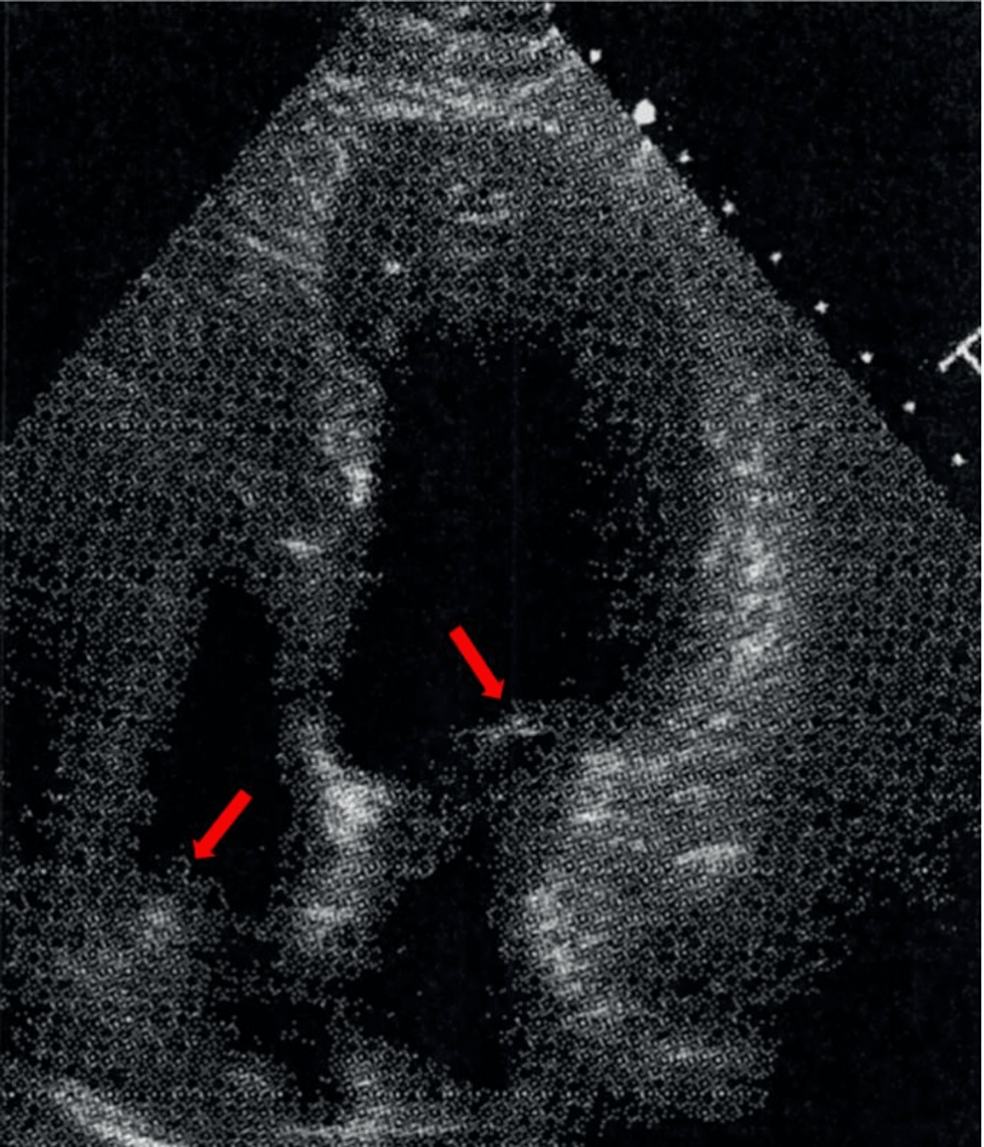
An echocardiogram image of the patient with mildly enlarged left ventricular (LV) wall thickness and pronounced trabeculation (shown in red arrows).
